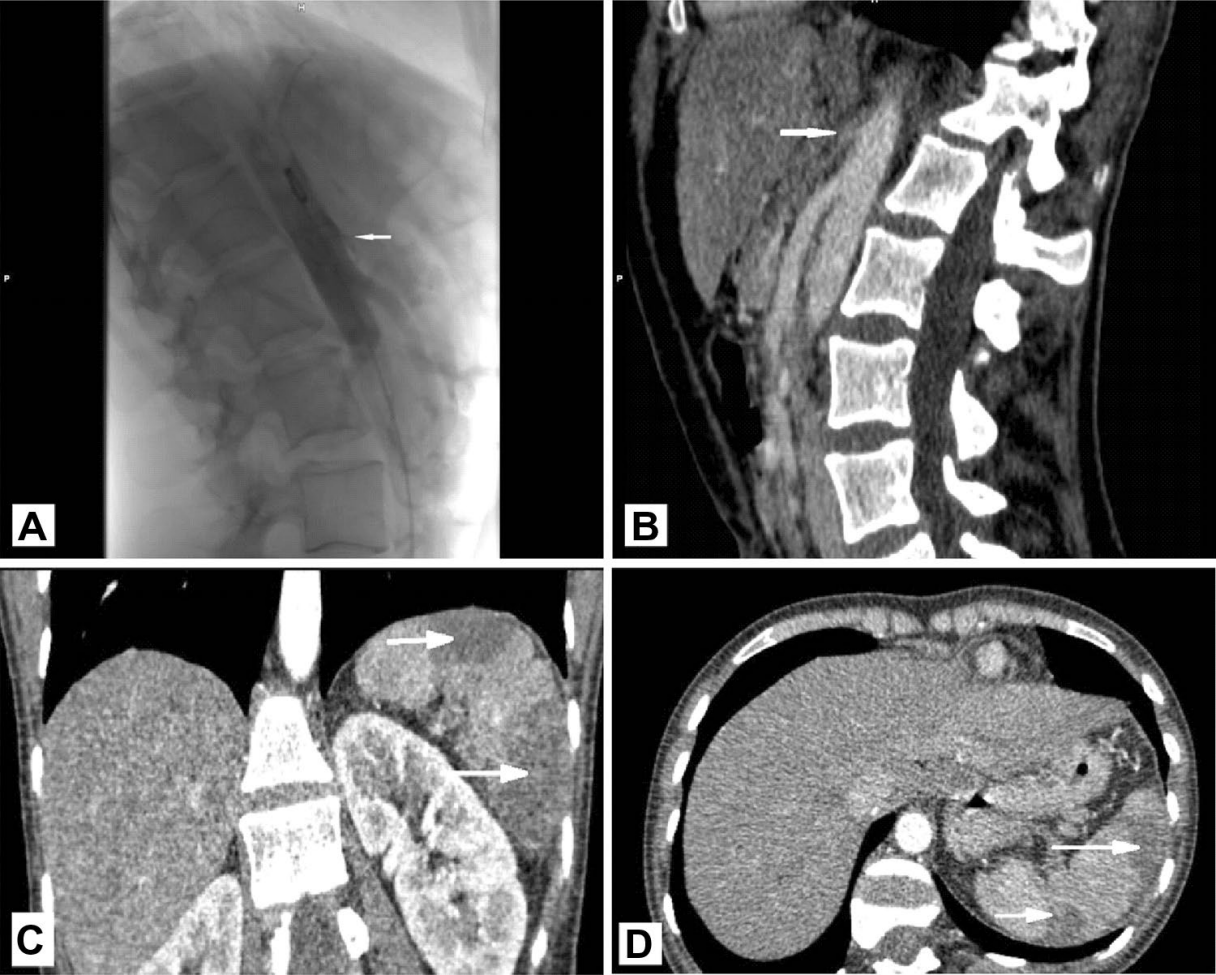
A Digital subtraction angiography (DSA) in sagittal plane showing compression of the celiac artery (arrow), B contrast enhanced CT image of aorta in sagittal plane showing compression of the celiac artery (arrow), C contrast enhanced CT image in sagittal plane showing spleen infarction (arrows), D contrast enhanced CT image in transverse plane showing spleen infarction (arrows)
